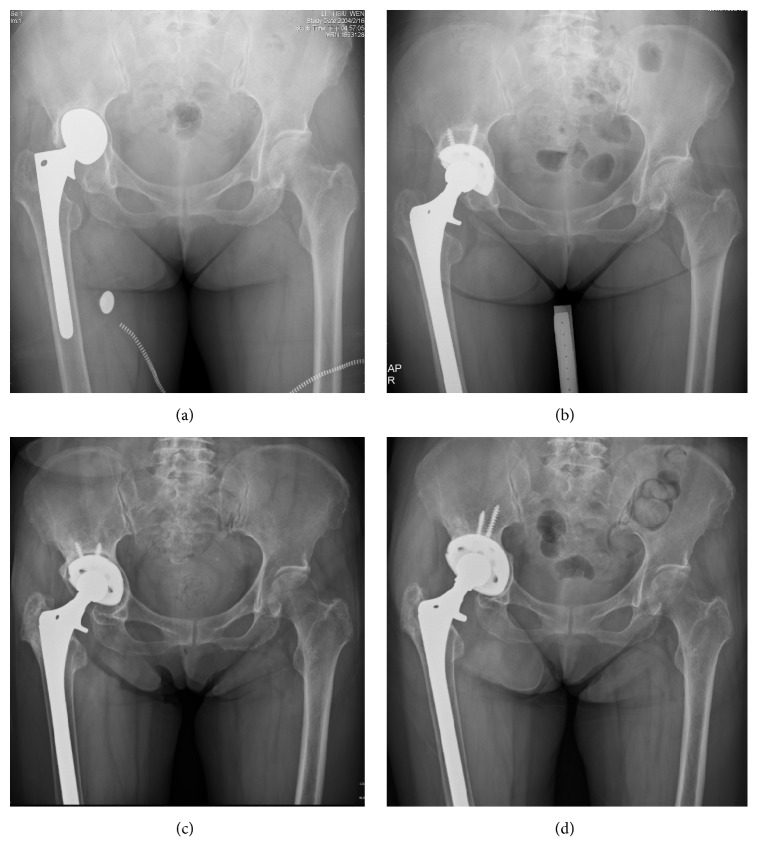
A 69-year-old female patient, with aseptic loosening of right bipolar hemiarthroplasty with acetabuli protrusio. (a) Preoperative radiographs showed up-and-in migration of the bipolar prosthesis with violation of Kohler's line (Paprosky IIIB). (b) The acetabular component was revised with structural and morselized allograft and Trilogy cup. (c) Loosening of the acetabular component at 7 years after 1st revision THA. (d) The 2nd revision was undertaken by allogenous bone grafting again and a titanium porous revision cup. Radiography at 6 years showed stable cup position and good incorporation of allograft.
